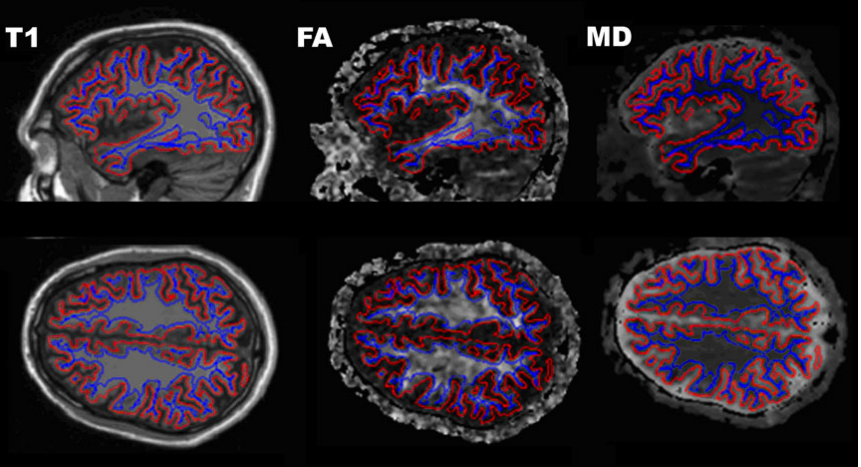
Quantification of tissue properties in the cerebral cortex and in pericortical white matter. Two surfaces were defined from the T1-weighted anatomical images (left column): in mid-gray matter (red) and 2 mm below the gray-white matter boundary (blue). White matter fractional anisotropy (middle) was quantified 2-mm below the gray/white boundary and mean diffusivity (right) was quantified in both the cortical gray matter and the white matter surfaces. Parasagittal (left hemisphere) and axial (above the lateral ventricles) cross-sections from the TBI patient's first imaging session are shown. High intensity regions on the FA map correspond to major fiber bundles running parallel to the cortical surface. Cerebrospinal fluid surrounding the cortex appears bright on the MD map.
